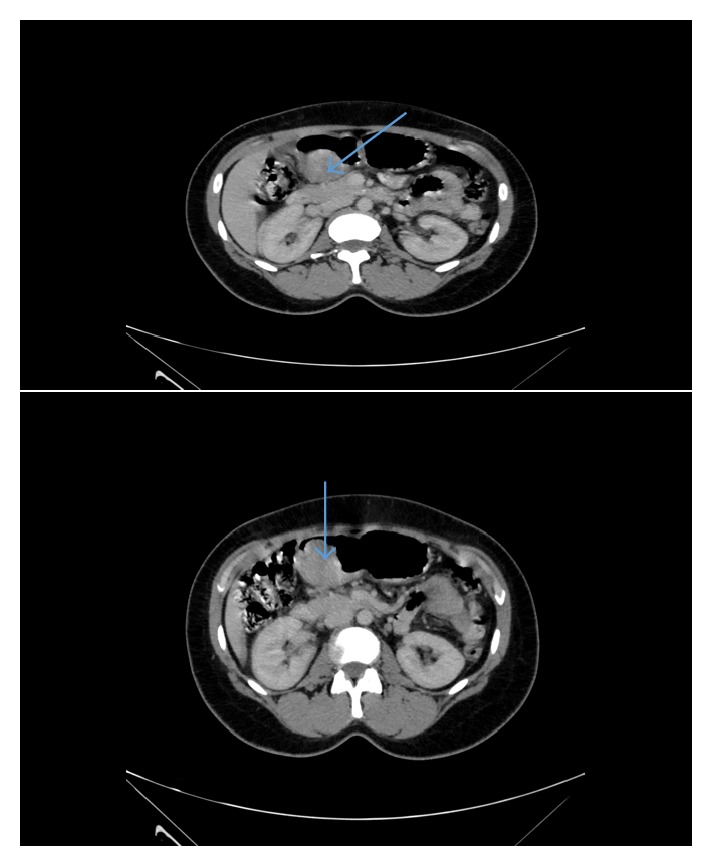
Axial images of CT scan of abdomen revealing mass in gastric antrum near pylorus.
